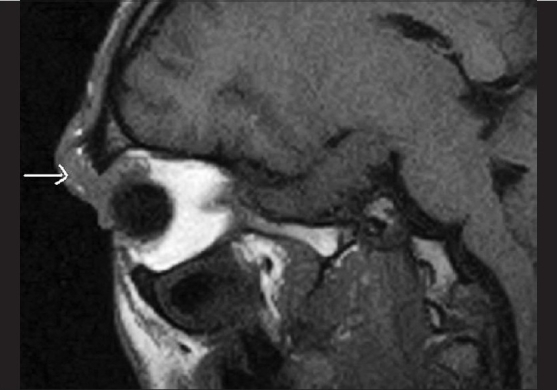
MRI sagital T1 showing soft tissue swelling in right periorbital region
